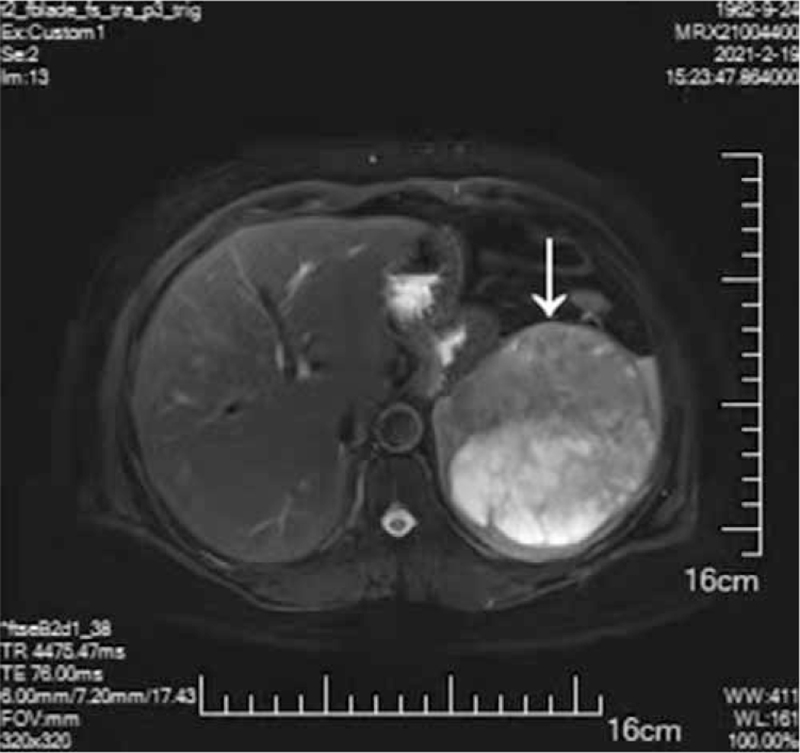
MRI image of the liver and spleen shows a space-occupying lesion of the spleen. MRI = magnetic resonance imaging.
